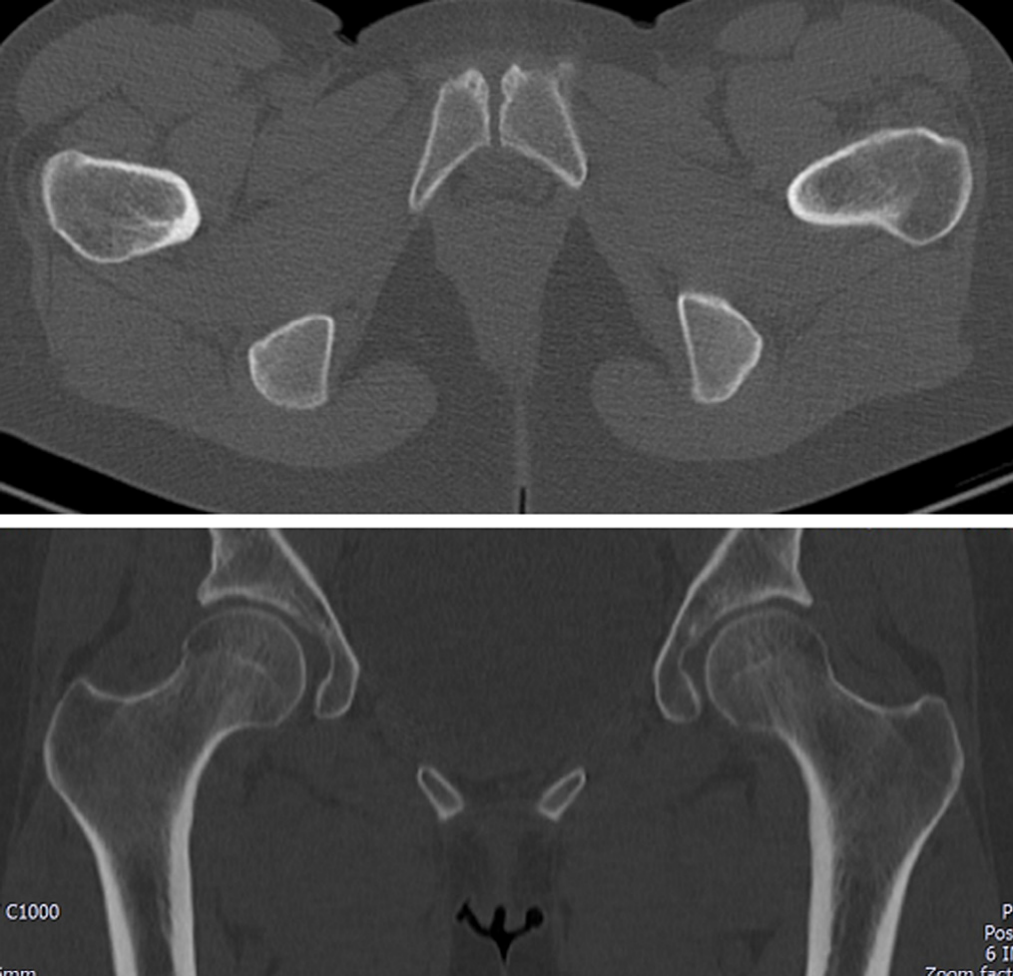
Pelvic CT-scan (A – axial view, B − coronal view) at twelve months, showing fracture union and no signs of complications.
